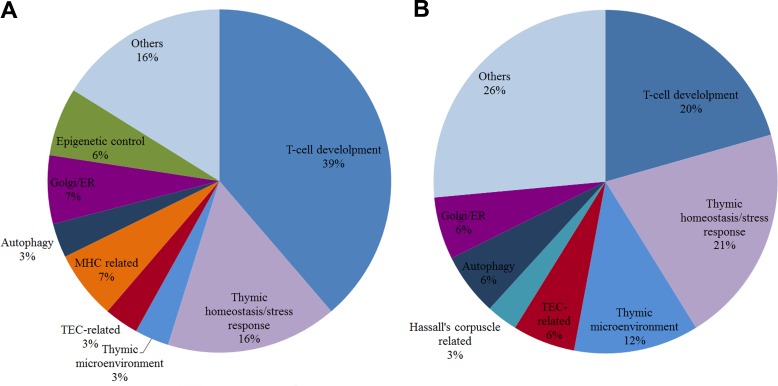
Pie chart of biological functions for high-hierarchy genes in DE networks Pie chart of HH gene functions in CT-DE network (A) and DS-DE network (B). Each slice represents the percentage of genes in a functional category.
